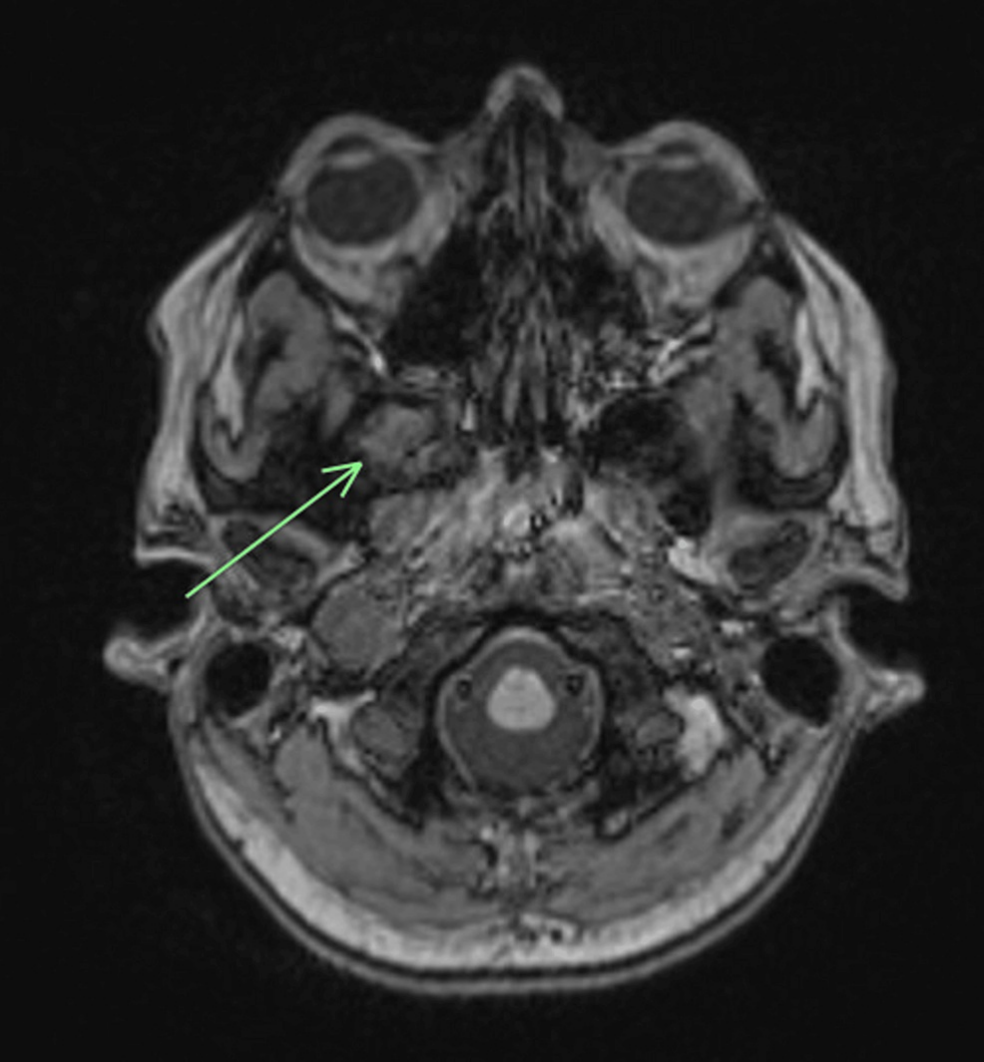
A T1-weighted MRI with contrast shows the known right temporal encephalocele (green arrow).
